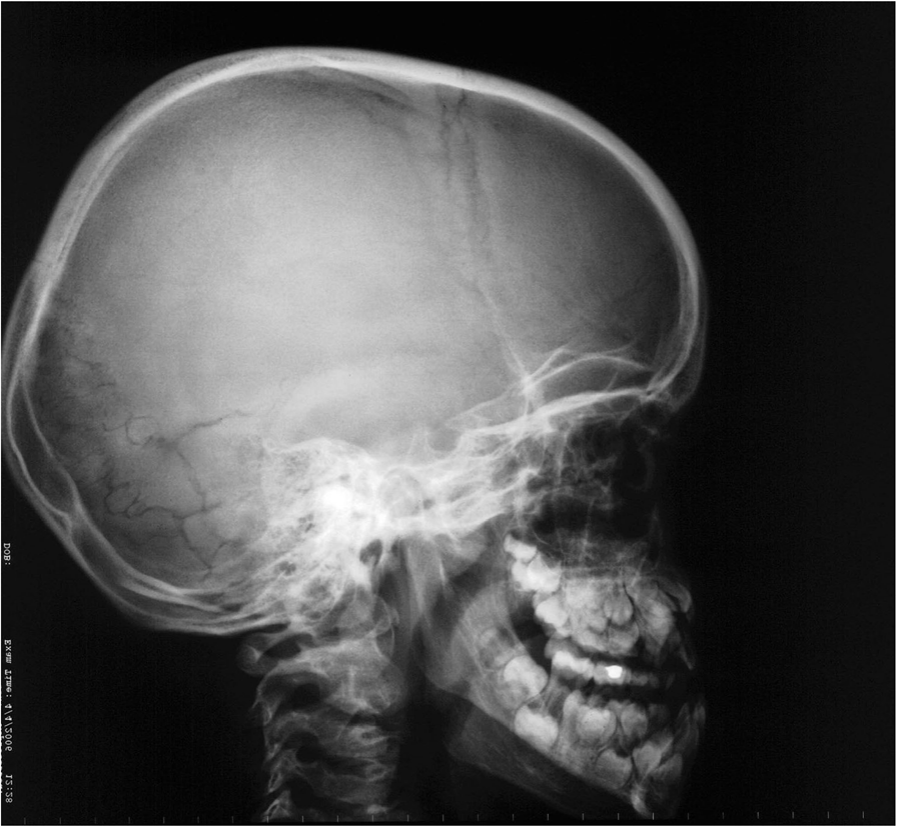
Lateral cephalometric X-ray showing numerous Wormian bones, large fontanelles, hypoplastic maxilla, and prognathic mandible. (From Kolokitha OE, Papadopoulou AK [1] with permission).
